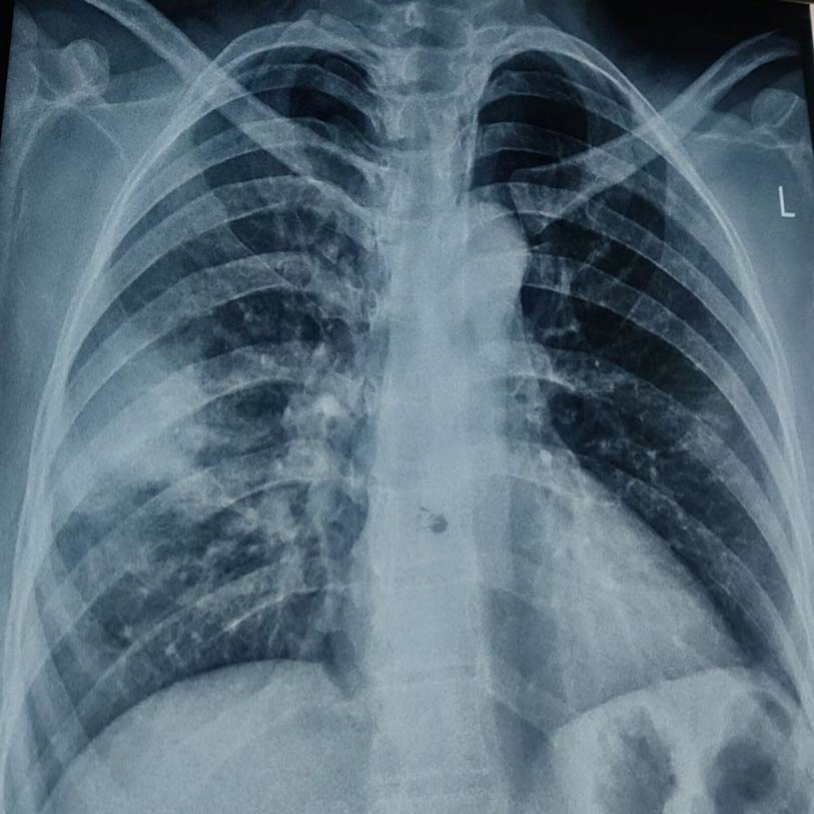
Chest X-ray posteroanterior view showing well-defined radiopaque mass with circumscribed medial margin in the peripheral aspect of the right middle zone.
